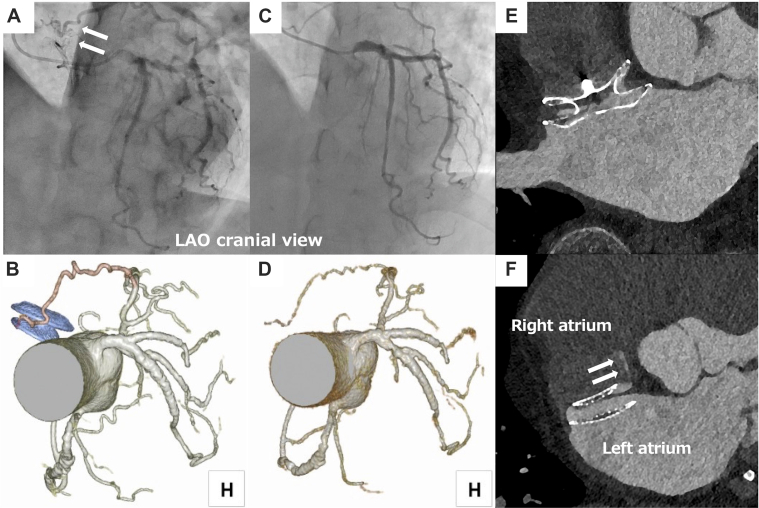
Findings on Coronary Angiography and Computed Tomography Coronary angiography (CAG) after atrial septal defect closure showed a collateral artery inside the device from the atrial branch of the left circumflex artery, highlighted by the white arrows in (A). The finding was observed on the contemporaneous computed tomography (CT) images (B), but had not been identified on previous CAG and CT images (C and D). In addition, CT showed contrast opacification within the device (E) and contrast leakage from the left to the right atrium, highlighted by white arrows in F.
